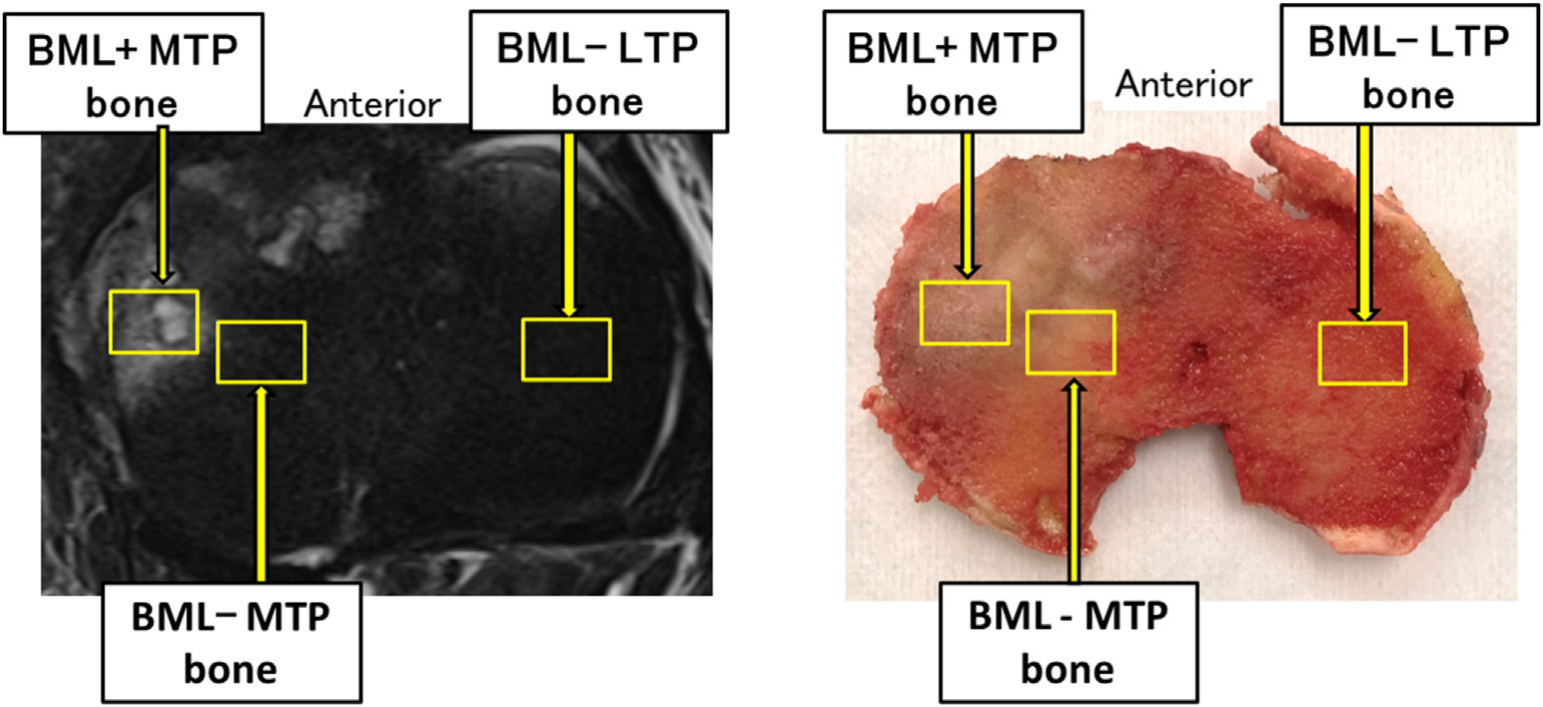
Co-localization of MRI Images and joint tissue obtained at surgery. Representative images of axial views of target knee for co-localization of knee biopsy tissue by MRI identification before tissue harvest at joint replacement surgery. Yellow boxes represent BML^+^ MTP bone, BML^−^ MTP bone and BML^−^ LTP bone that were analyzed. MTP: medial tibia plateau, LTP: lateral tibial plateau.
